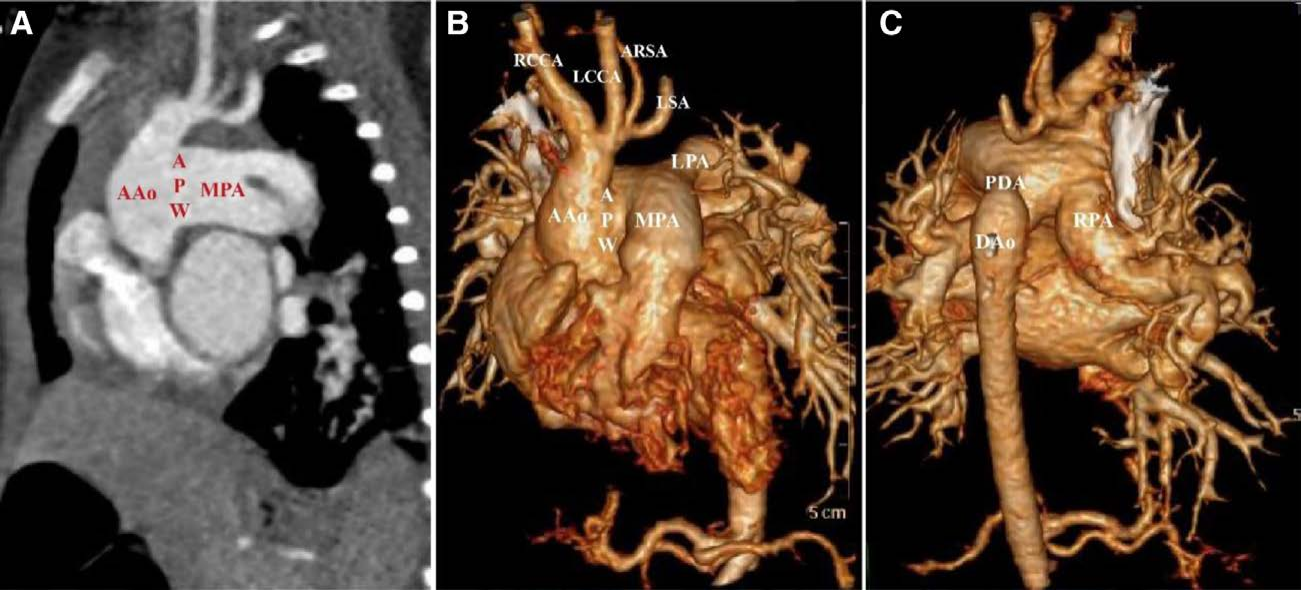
(A) CTA revealed a large aortopulmonary window between ascending aorta and main pulmonary. (B) Three-dimensional reconstruction demonstrated type A interrupted aortic arch and 4 branches of aortic arch. (C) Three-dimensional reconstruction revealed aortic origin of the right pulmonary artery and a large patent ductus arteriosus. CTA = computed tomography angiography.
